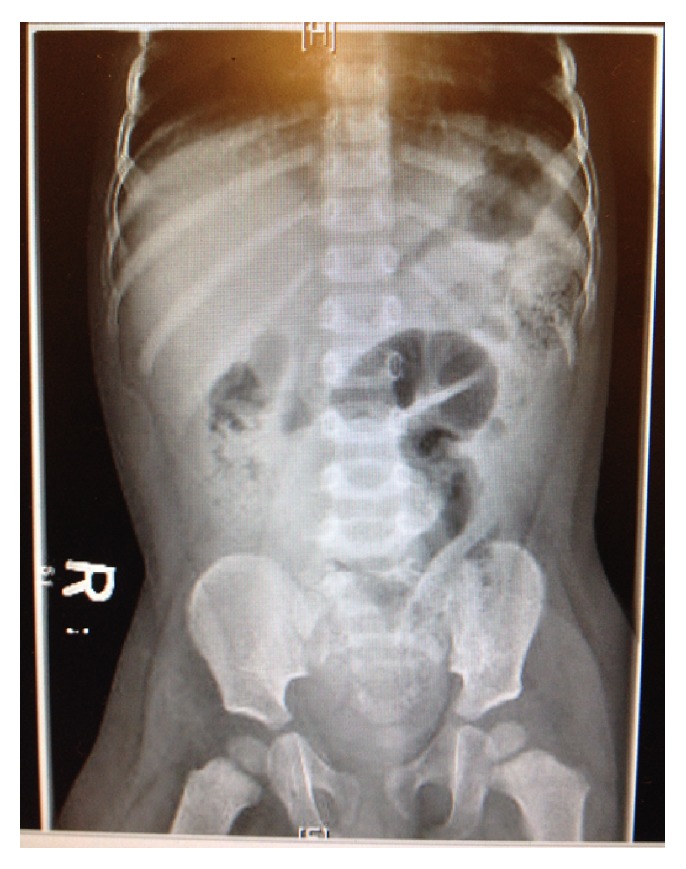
Supine abdominal plain film.
